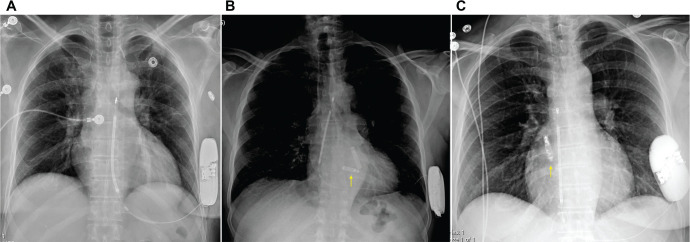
A: Frontal chest radiograph of a subcutaneous implantable cardioverter-defibrillator (S-ICD). B: An S-ICD system that includes a leadless pacemaker in the right ventricle (arrow) with the ability to deliver anti-tachycardia pacing. Note there is also an implantable cardiac rhythm therapy device. C: An S-ICD system and a separate atrial AVEIR device.
